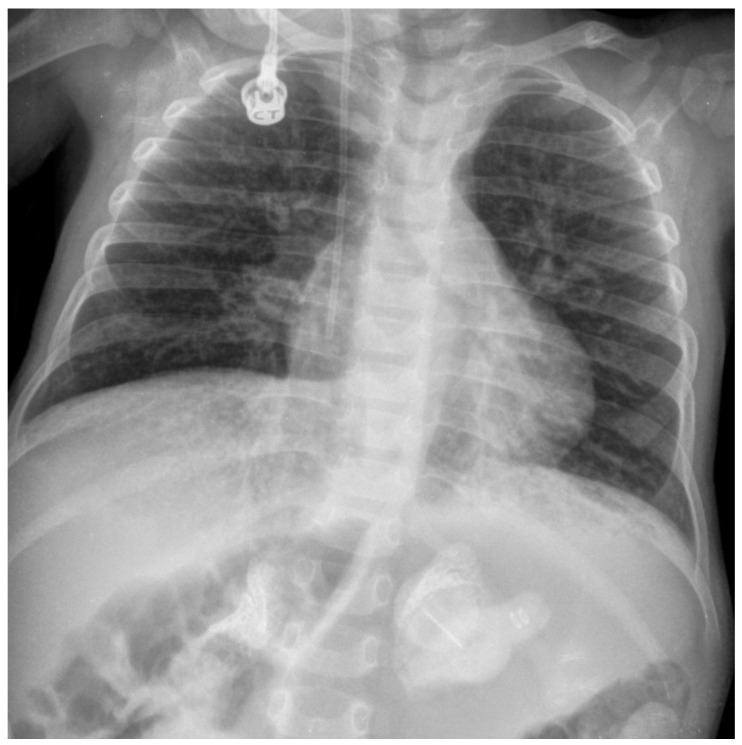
Abdominal X-ray showing adrenal gland calcifications in a patient with infantile-onset lysosomal acid lipase deficiency (LAL-D). Image courtesy of Dr. de las Heras.
